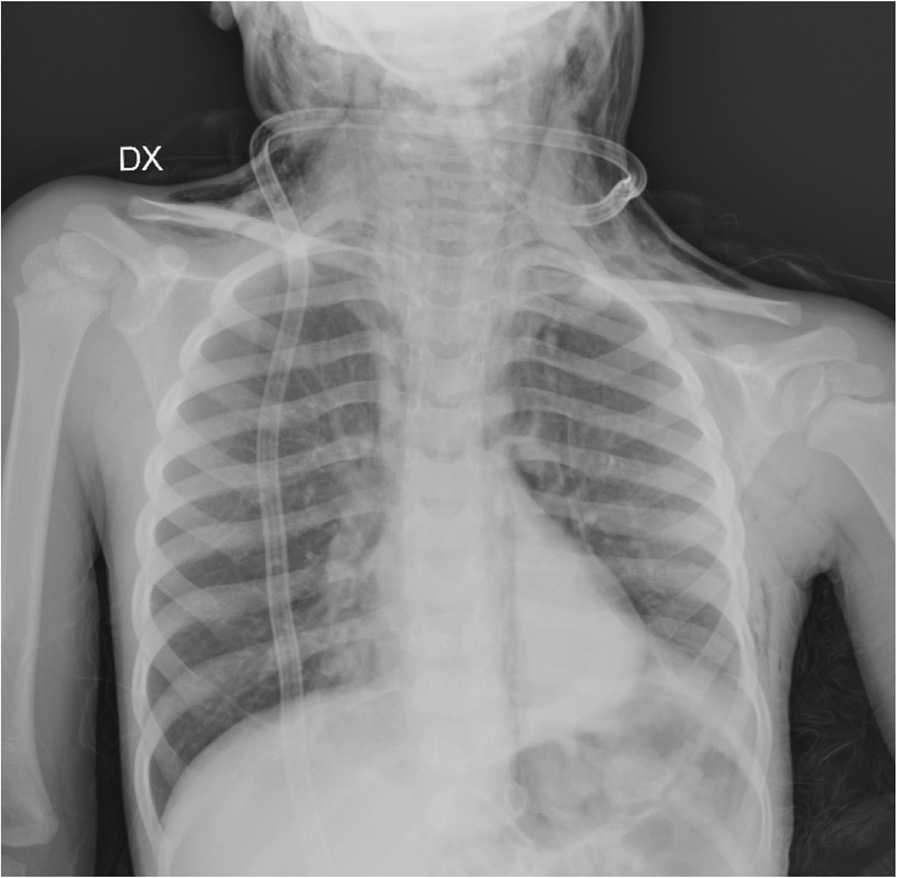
Chest X-ray examination revealed the presence of diffuse subcutaneous emphysema of the neck extending along the left lateral chest wall. Pneumomediastinum was also appreciable: air within the superior mediastinum on both sides and along the left paravertebral space. A loss of volume of the left lung due to left inferior lobe atelectasis was associated with the elevation of the left diaphragmatic dome
